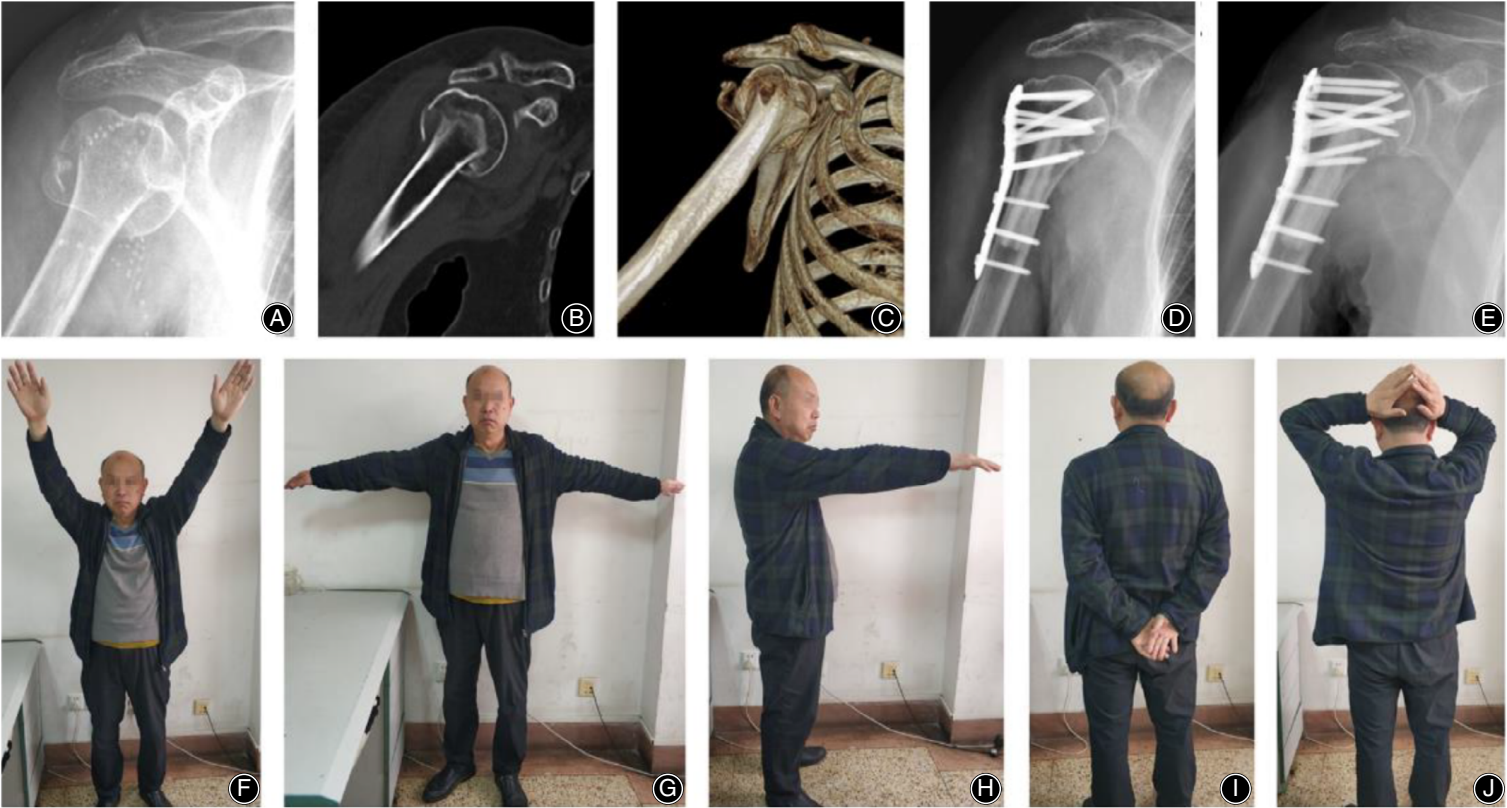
PHILOS fixation with fibular allograft in a 76‐year‐old male patient with a four‐part proximal humeral fracture on the right side. (A) X‐ray film before surgery; (B) CT before surgery; (C) 3D CT before surgery; (D) X‐ray film after surgery; (E) X‐ray film 3 months after surgery; (F–J) Function of patient's upper arm at the last follow‐up.
